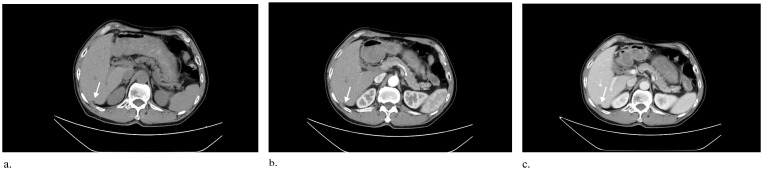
(a) Axial unenhanced CT image shows a 1.2x0.8 cm ovoid fat density nodule under the pericardium of the right posterior lobe of the liver (arrow). (b, c) Axial contrast-enhanced CT scans of the arterial and portal venous phases show no enhancement of the fat-dense nodule (arrows), and the nodule is accompanied by peripheral calcification in the portal venous phase.
